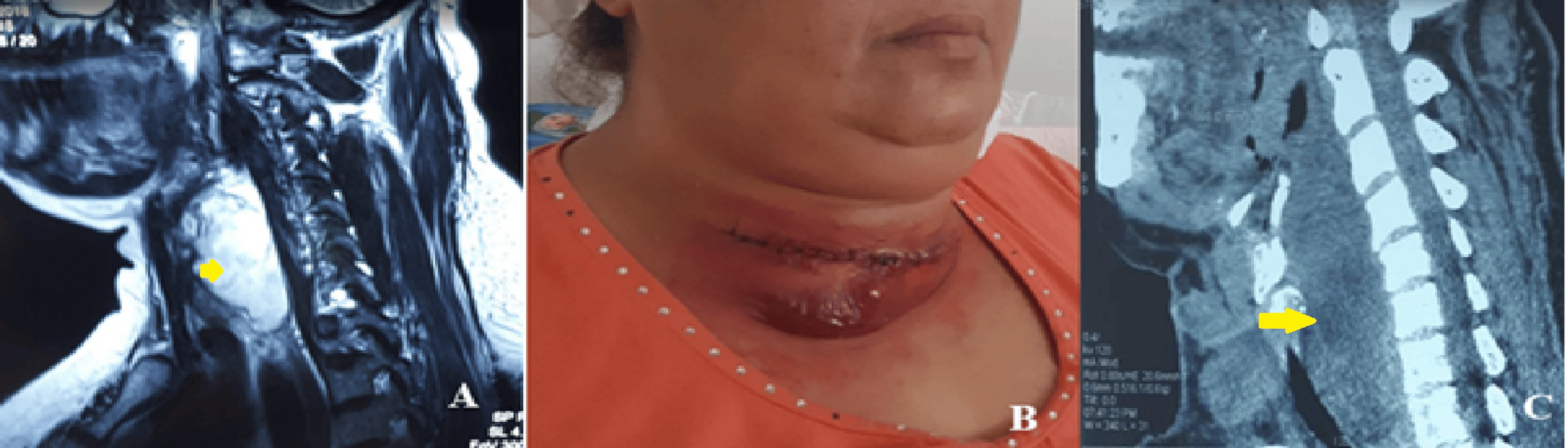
Case of a 42-year-old woman with a cervical synovial sarcoma. (A) Sagittal MRI showing a well-defined retroesophageal mass (yellow arrow) in a patient with a history of papillary thyroid carcinoma, initially suggestive of metastatic recurrence. (B) Rapid tumor progression after surgical exploration and biopsy. (C) Sagittal postoperative CT scan showing a poorly defined, infiltrative tumor (yellow arrow).
